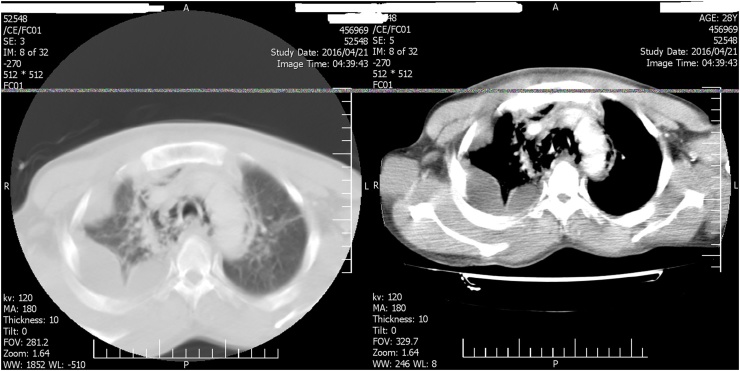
Axial lung and mediastinal window CT scan, show loculated pleural effusion and pleural enhancement due to empyema in right hemi thorax.
